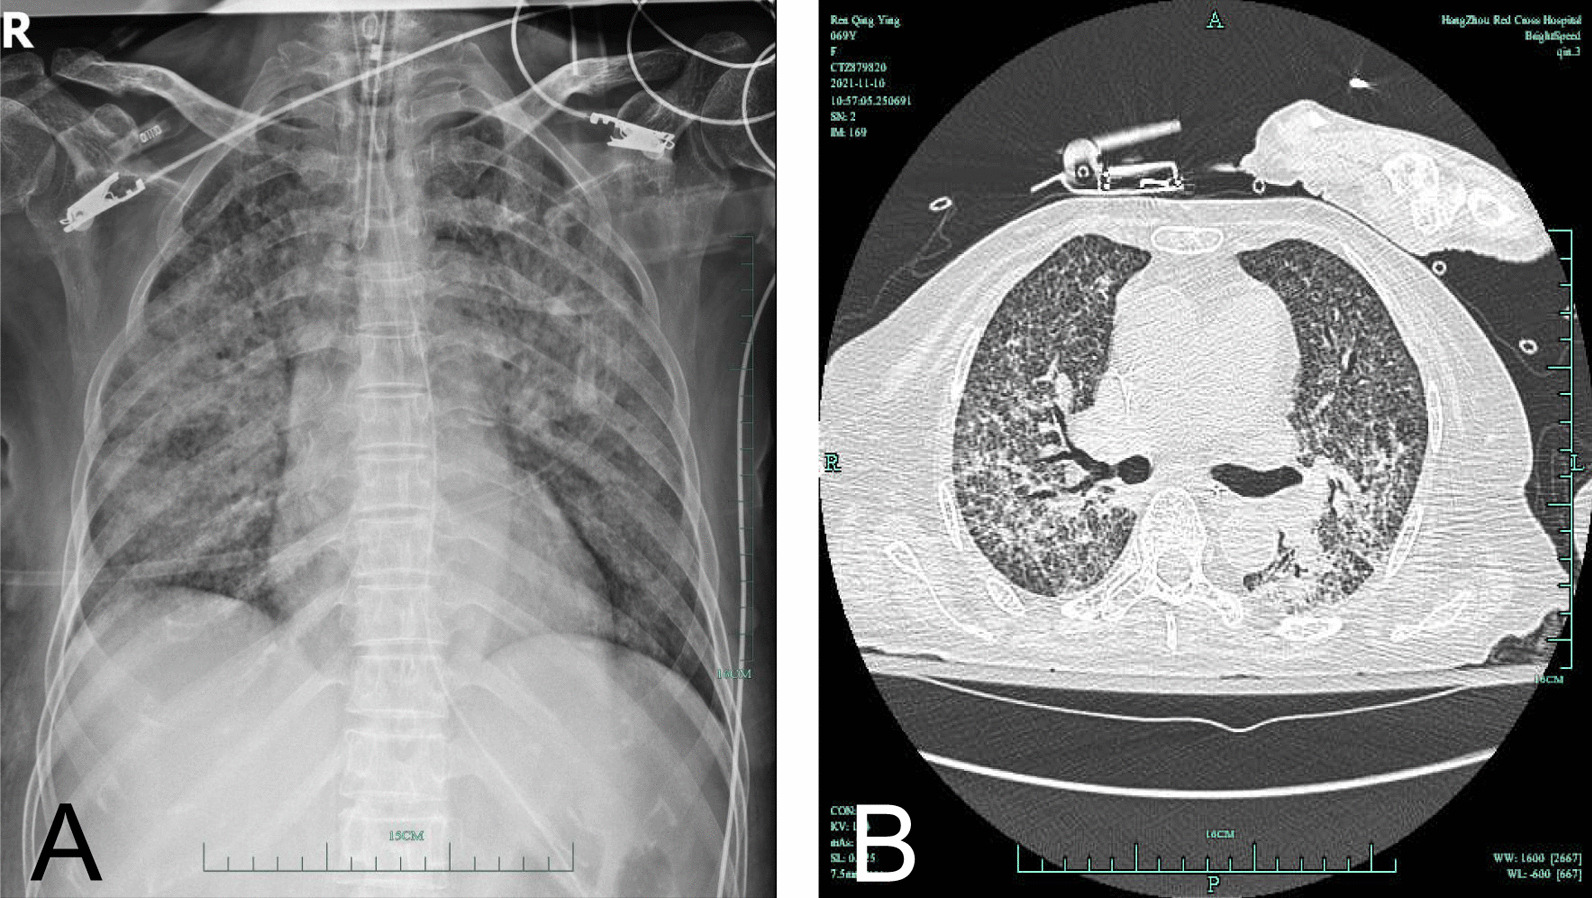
Chest imaging findings during the hospital admission. A The X-ray shows increased bilateral lung markings with diffuse patchy infiltration. B The computed tomography scan shows bilateral diffuse patchy miliary nodules
